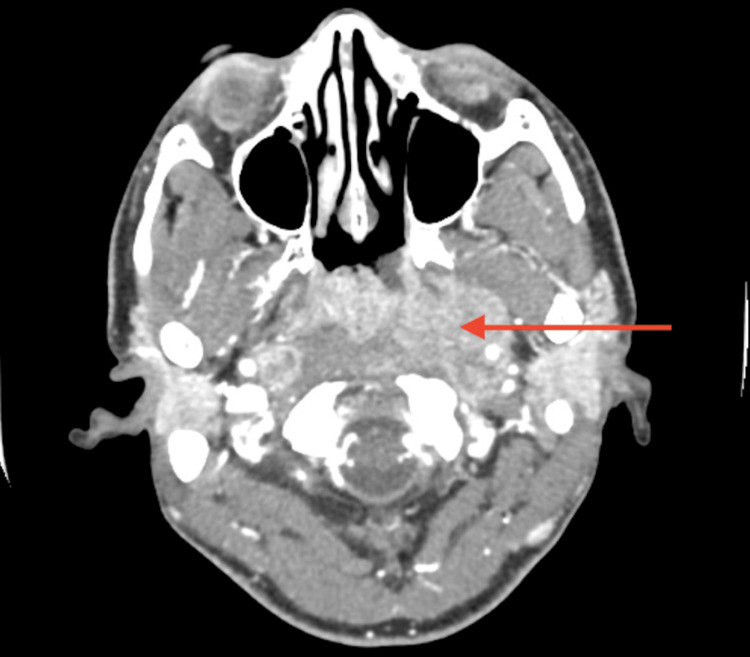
Head CT (axial plane, without contrast) revealing a left-sided nasopharyngeal soft tissue mass (arrow) CT, computed tomography
